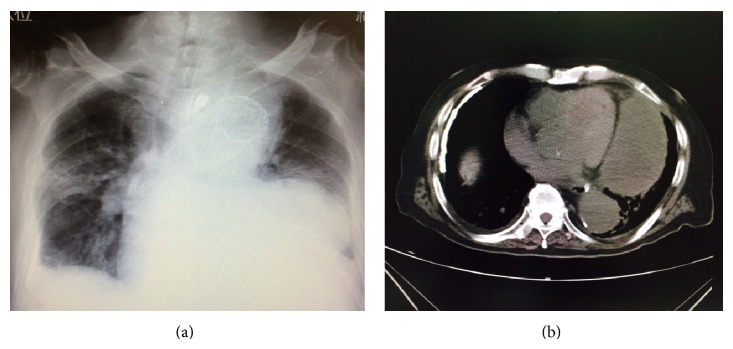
(a) Plain radiograph taken at postoperative day 25. (b) Image from plain CT performed at postoperative day 20. Resorption of the hematoma had progressed, and its size had decreased. The findings revealed that hemostasis was achieved as a result of TEVAR and that the patient's condition tended to improve.
